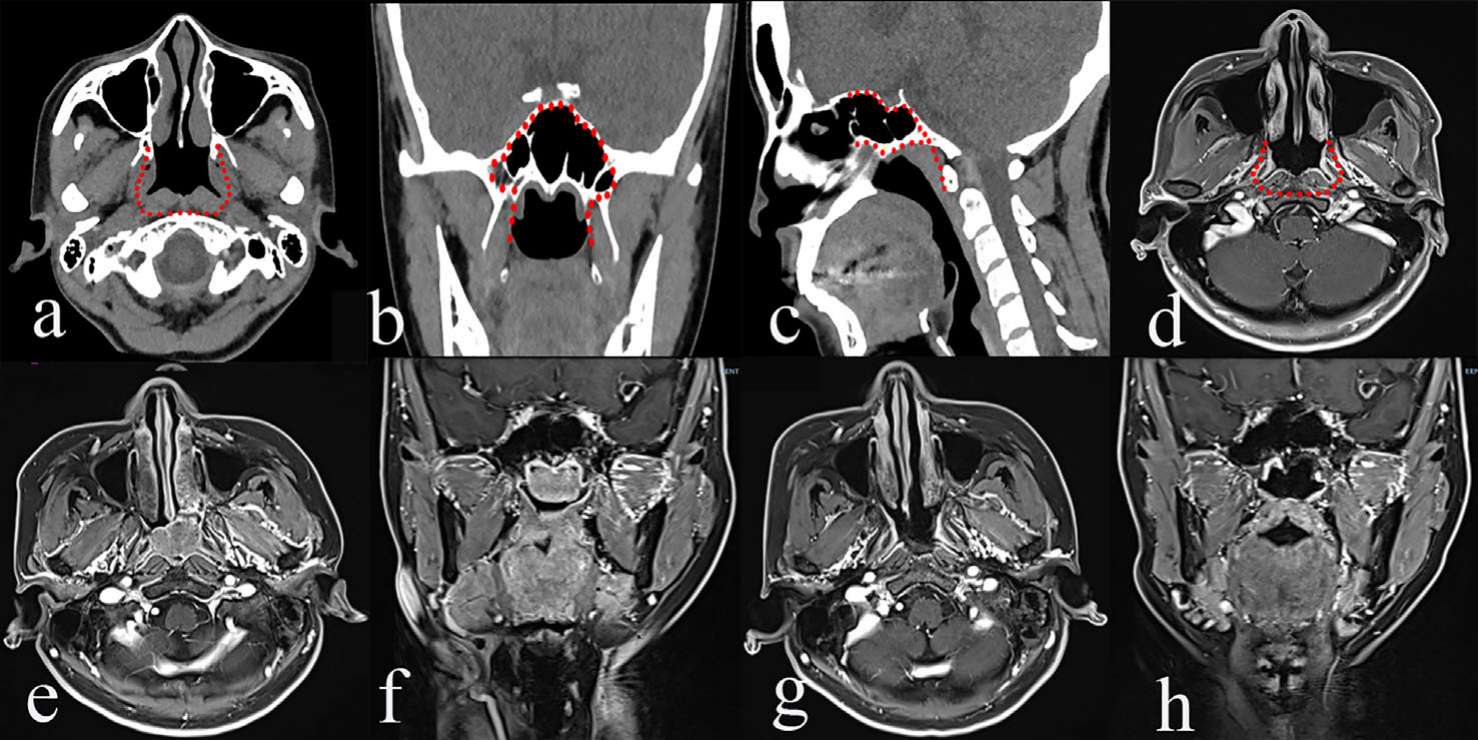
The greatest extent of resection in Type I transnasal endoscopic nasopharyngectomy (TEN) [red dot line in a–d], pre-operative magnetic resonance imaging (MRI) of rT1 nasopharyngeal carcinoma (NPC) (e, f), and post-operative MRI (g, h).
